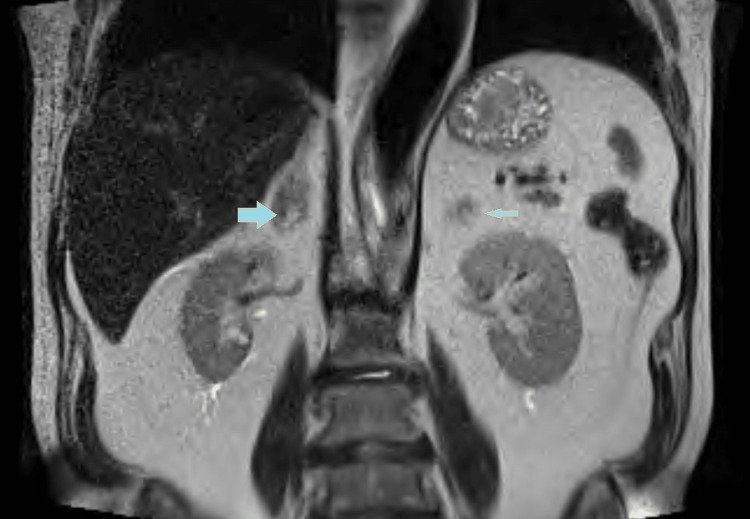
MRI of the abdomen (coronal section) showing right and left adrenal gland hemorrhage (Blue arrow with yellow margin).
